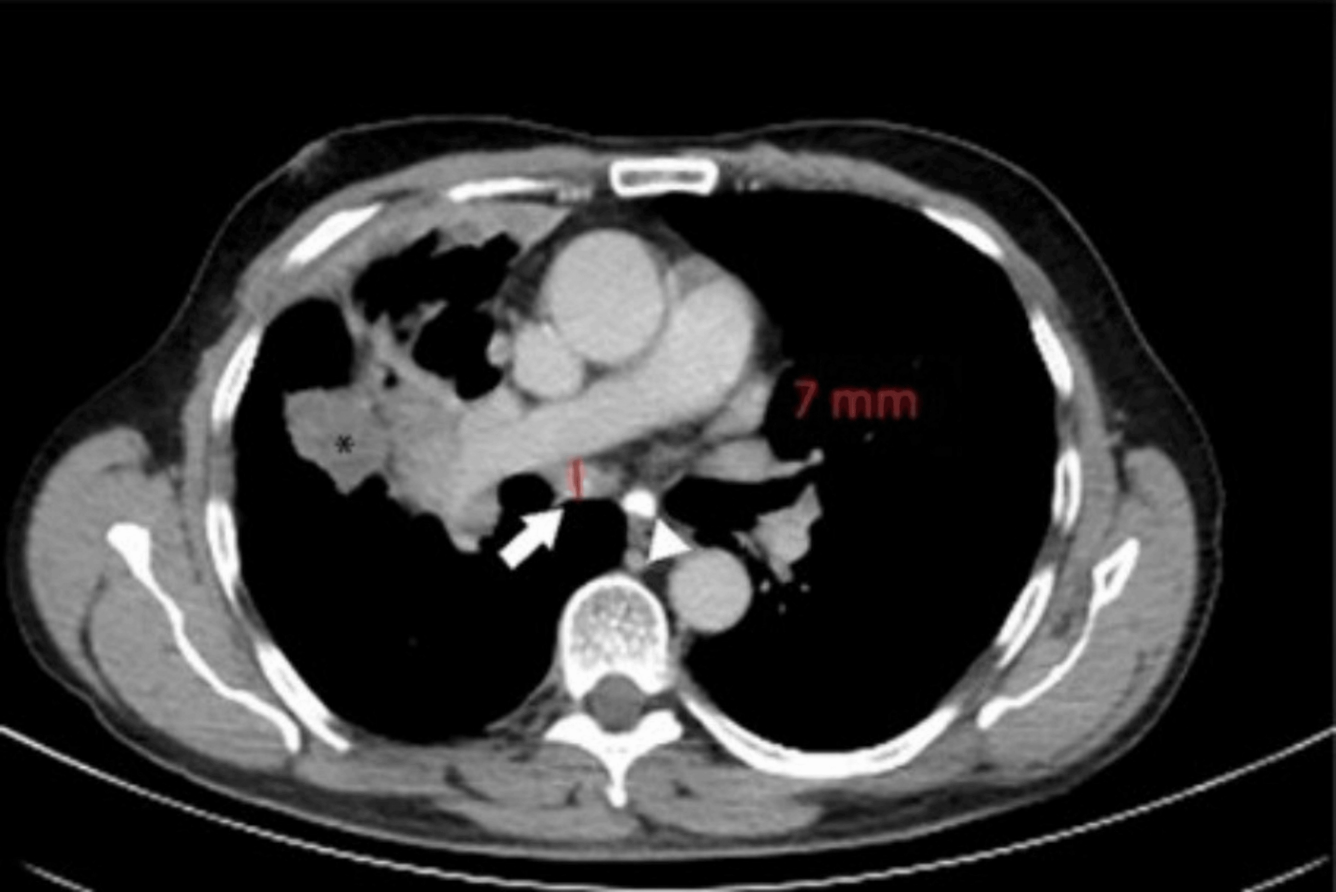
CT component showing a large heterogeneous solid mass in the right lung perihilar location, a prominent subcarinal node measuring 7 mm in short-axis dimension (arrow), and nodal calcifications in another subcarinal lymph node (arrowhead) CT: computed tomography
